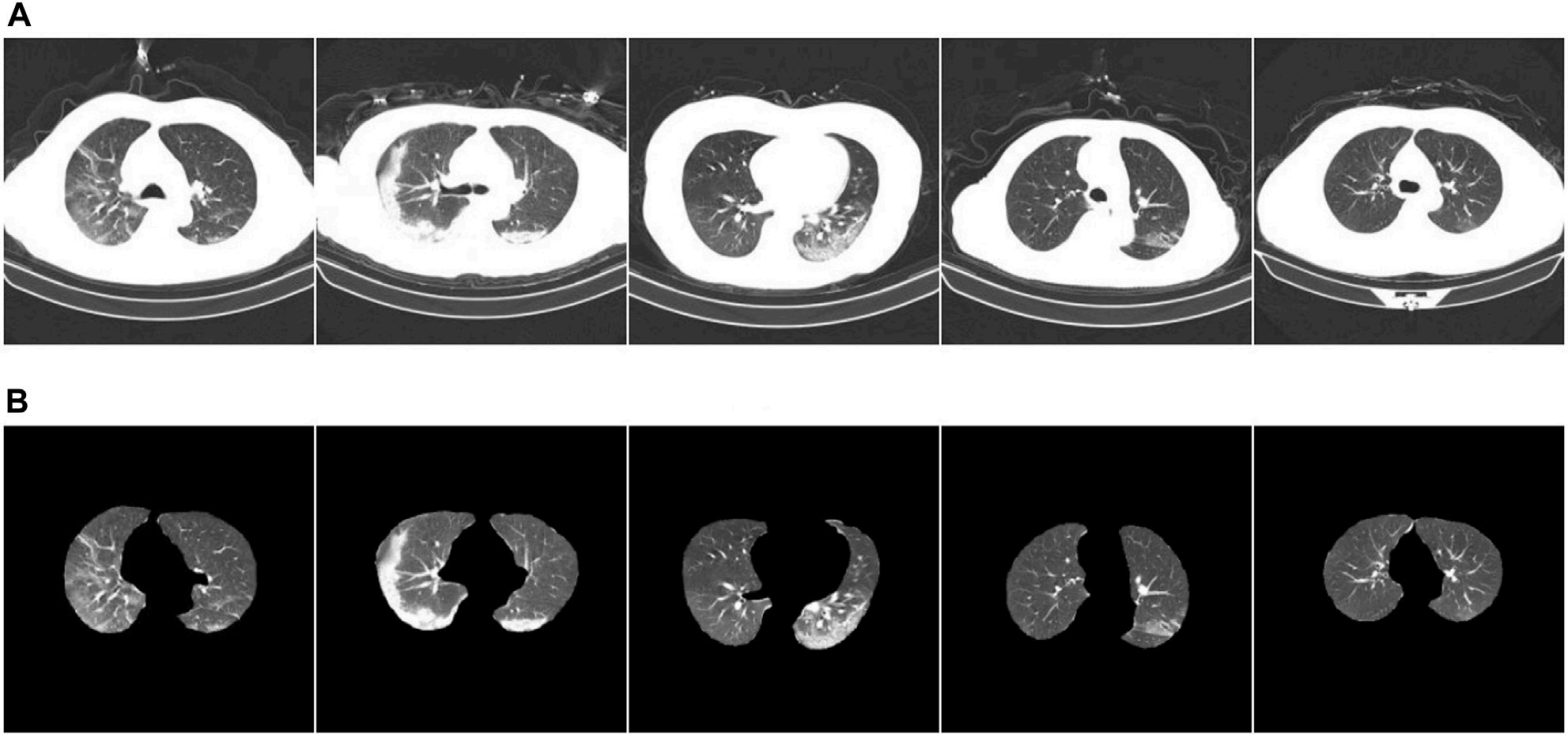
Example of ablation experiment comparison. (A) The CT image of the COVID-19 patient; (B) the result.
